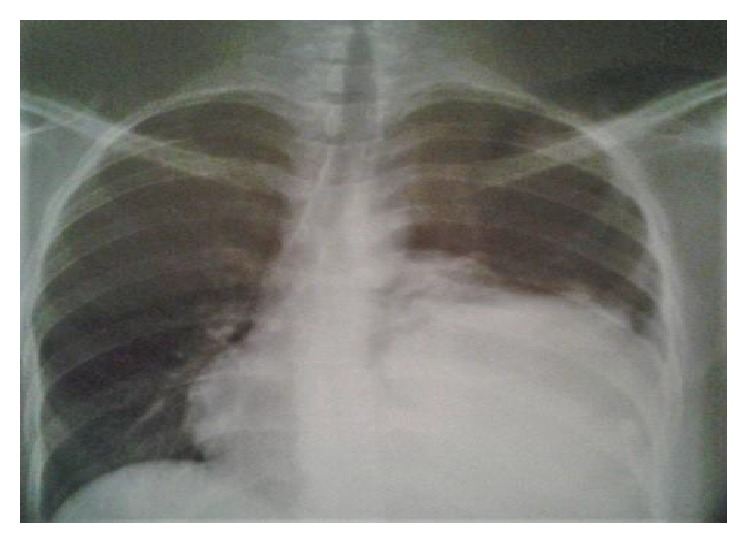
Chest X-ray shows opacity at the lower part of the left hemithorax.
